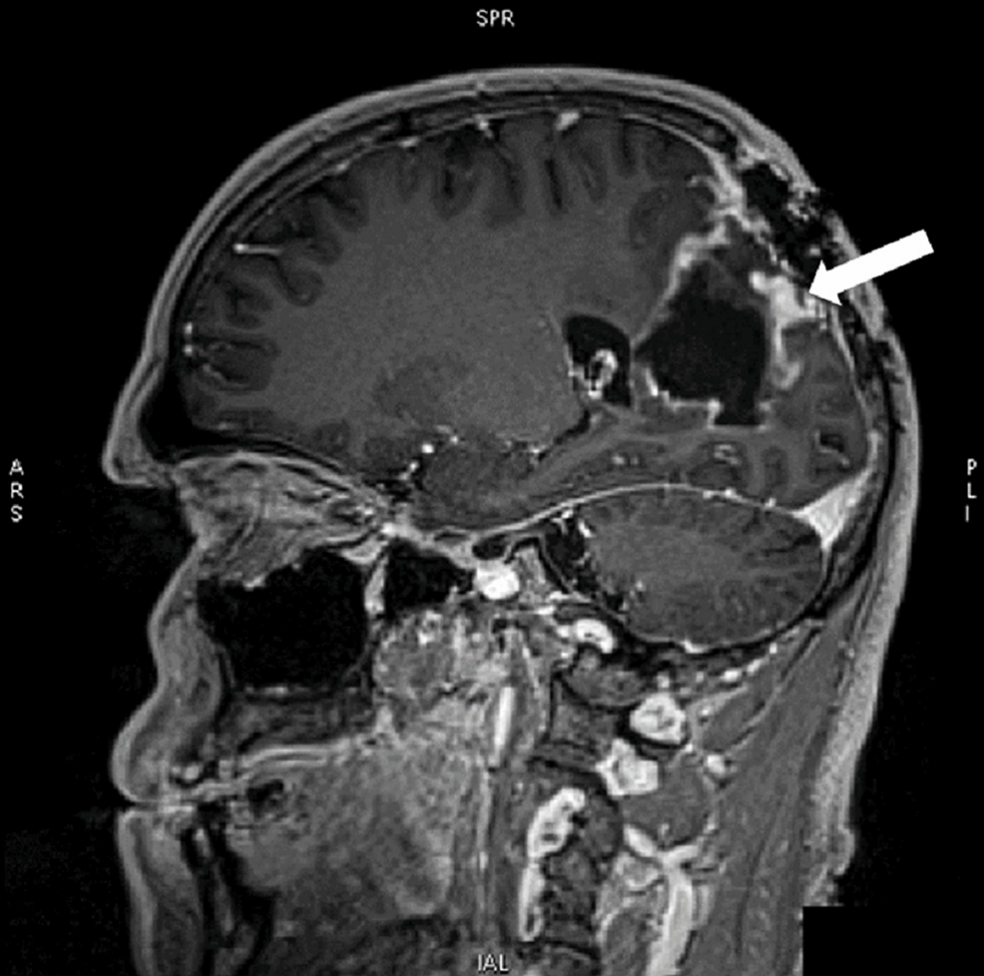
SAG 3D T1+C MRI of the brain showing postoperative changes with right parietal porencephalic cyst and peripheral zones of contrast enhancement, suggestive of residual tumor (arrow) SAG 3D T1+C MRI: sagittal three-dimensional longitudinal relaxation time contrast-enhanced magnetic resonance imaging
